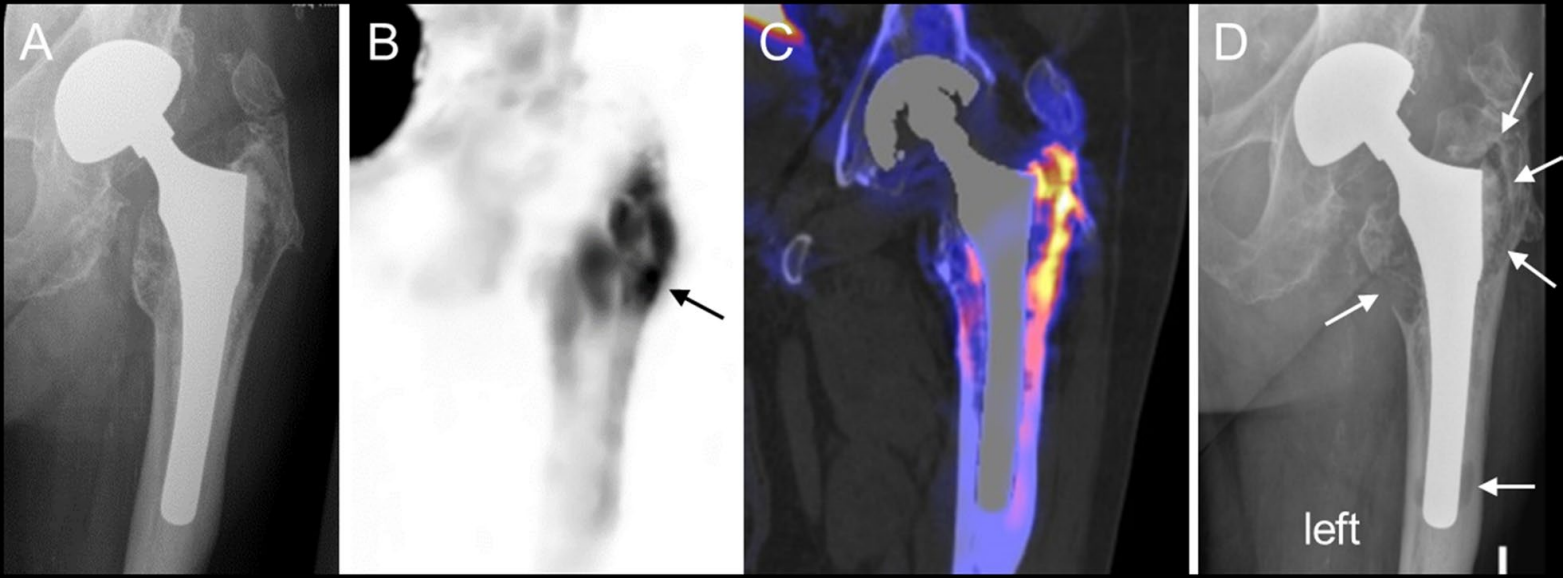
Same patient as in Fig. 1. a Plain radiograph at baseline without evidence for a loose prosthesis, b xSPECT Bone maximum intensity projection and c xSPECT Bone in combination with CT (CT with iMAR) at baseline as well. Periprosthetic SUVmax was 27.3 (black arrow) which was definitely above the cut-off value for a loose prosthesis (xSPECT Bone cut-off value: 14.78). Revision operation was refused. d Plain radiograph 2 years later showed a loose left prosthetic stem with progression of radiolucency around the femoral stem (white arrows) which correlates with pathological ^[99mTc]Tc−DPD^ uptake at baseline (b, c)
